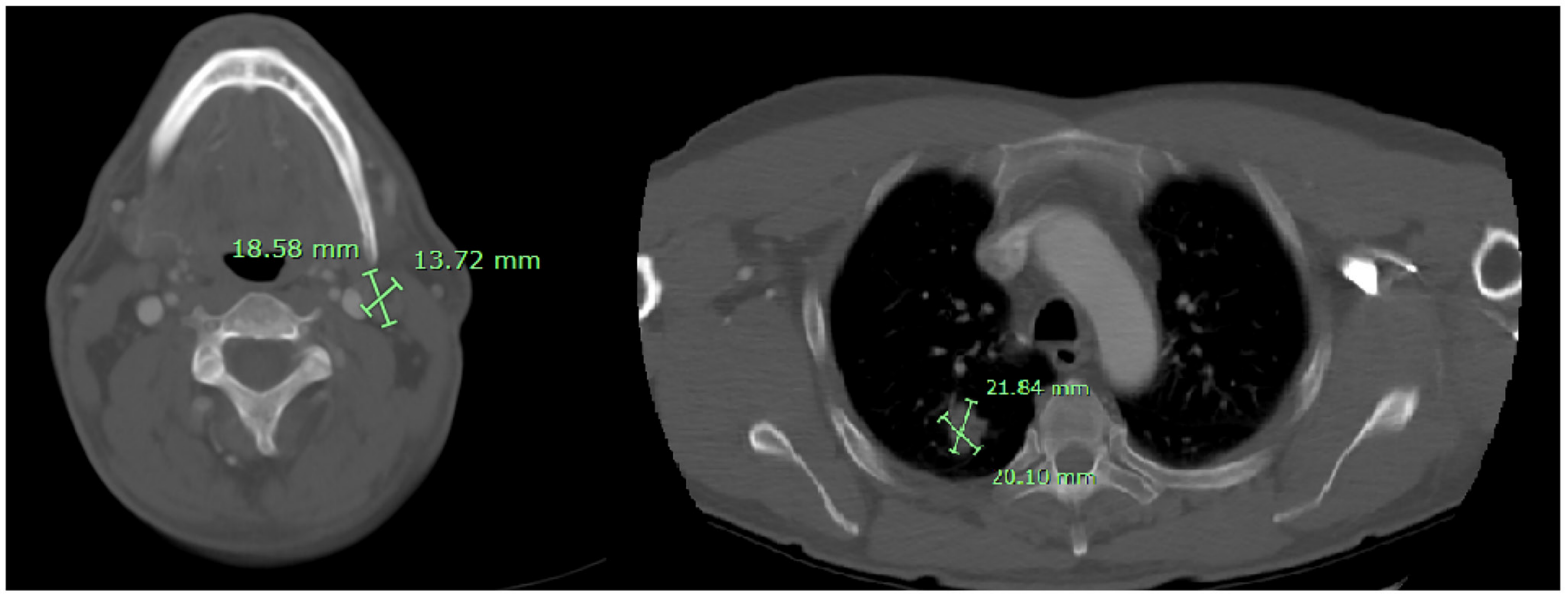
Axial contrast-enhanced CT scan at diagnosis. The image shows a carotid-jugular adenopathy (left), nodular spiculated lesion in the superior right lobe (right), dimensions in green mark.
